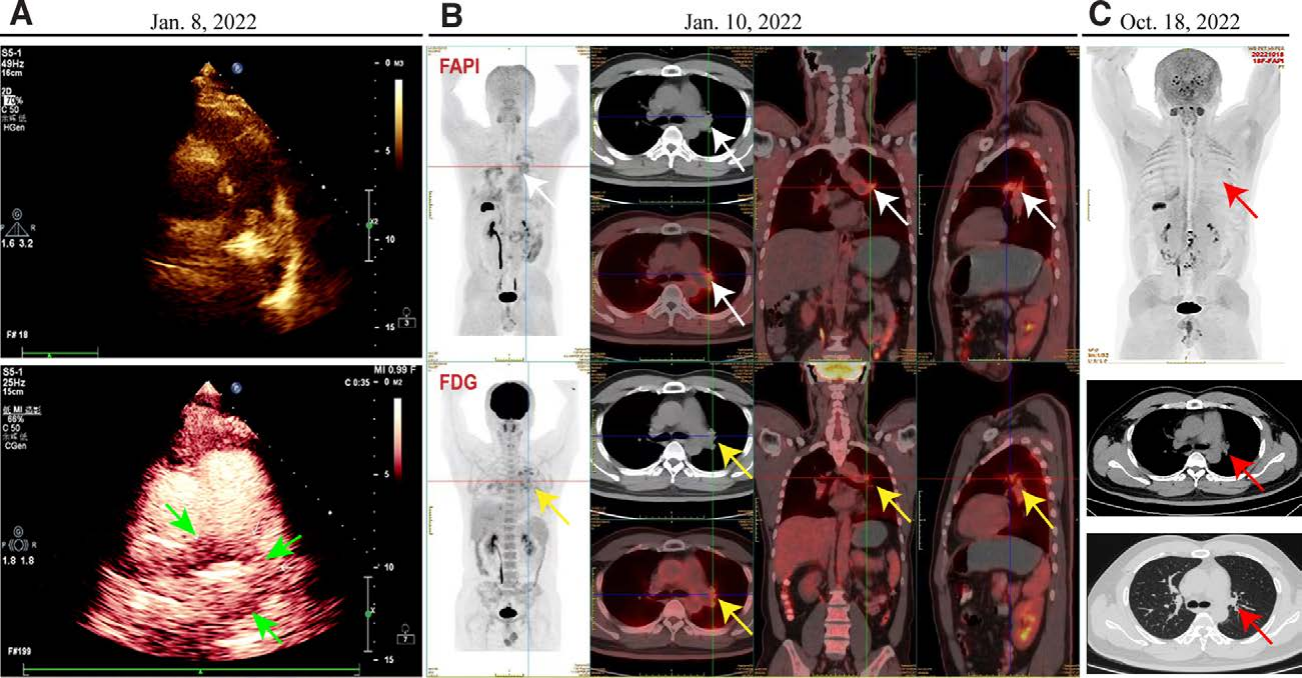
Visualization of the pulmonary endovascular lesions with ^18^F-FDG/FAPI-PET/CT and right heart contrast echocardiography. (a) right heart contrast echocardiography showed a solid mass with abundant blood provision in the pulmonary artery (green arrow). (b) Before diagnosing PE-EHE, ^18^F-FDG/FAPI-PET/CT presented an endovascular lesion with a SUVmax of 4.5 in the left main vessels in the 3-dimensional fluoroscopy, transverse, sagittal plane and coronal planes (from left to right, respectively). the white arrow represent the 18F-FAPI-PET/CT, and the yellow arrow for the 18F-FDG -PET/CT. (c) ^18^F-FAPI-PET/CT revealed the SUVmax of the left pulmonary vascular lesion reduced to the background level (red arrow). ^18^F-FDG/FAPI-PET/CT = 18-fluorodeoxyglucose/fibroblast associated protein inhibitor-positron emission tomography/ computed tomography, PE-EHE = pulmonary endovascular epithelioid hemangioendothelioma, SUVmax = maximum standardized uptake value.
